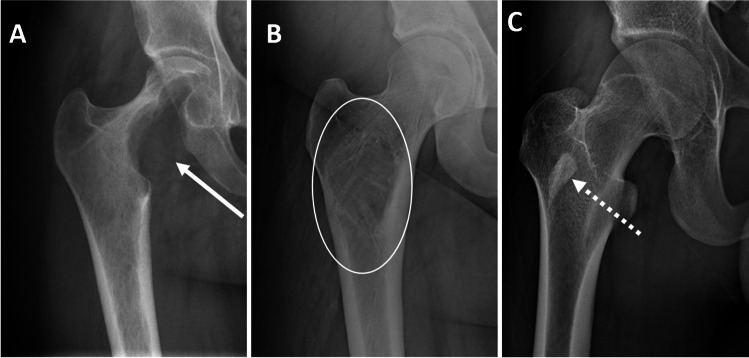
Radiographic examples of lucent (a arrow — giant cell tumor), mixed (b circled area — fibrous dysplasia), and sclerotic (c dotted arrow — bone island) of the proximal femur
